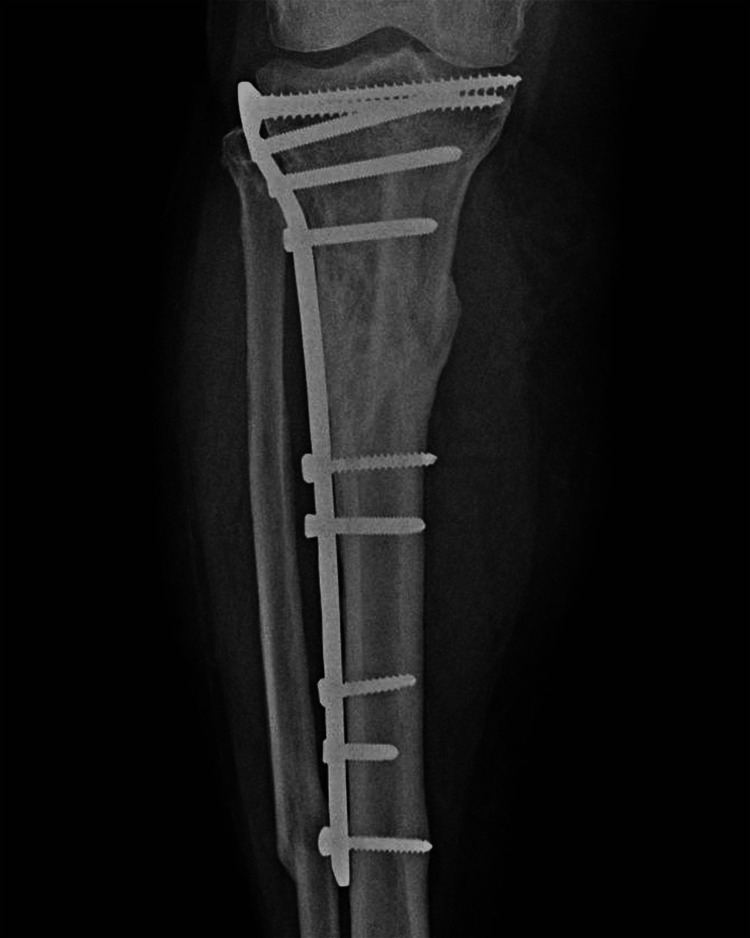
Anteroposterior X-ray of the right leg taken at a two-year postoperative follow-up, showing the bone union and the fibula shaft graft visible in the tibia canal
